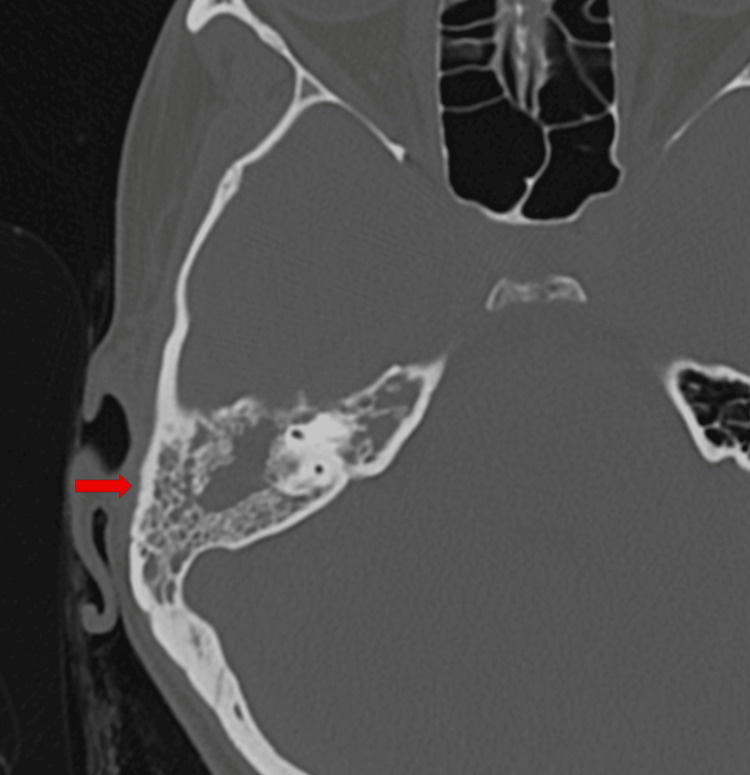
Ear CT scan evidencing complete opacification of the right mastoid cells (arrow). CT: computed tomography.
